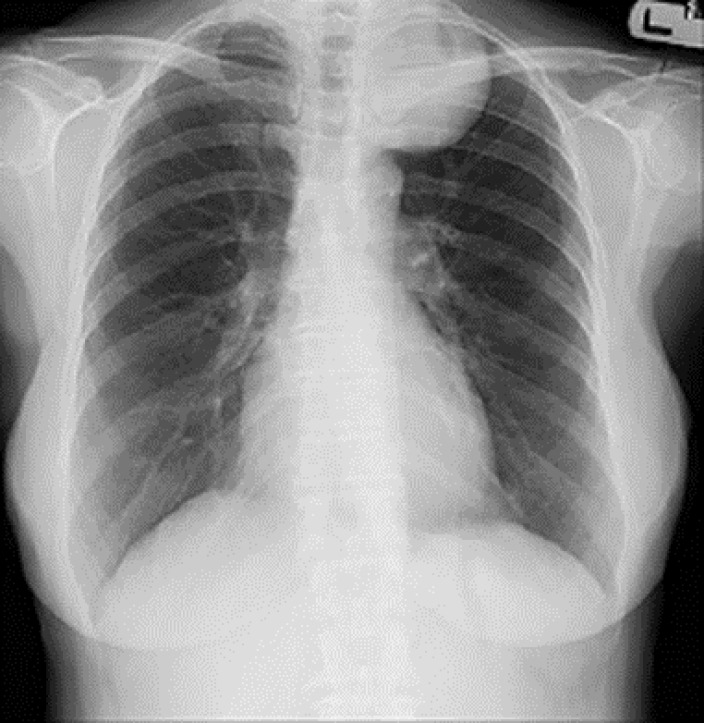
Chest radiograph showing a well-circumscribed spherical opacity occupying the upper part of the left hemithorax
